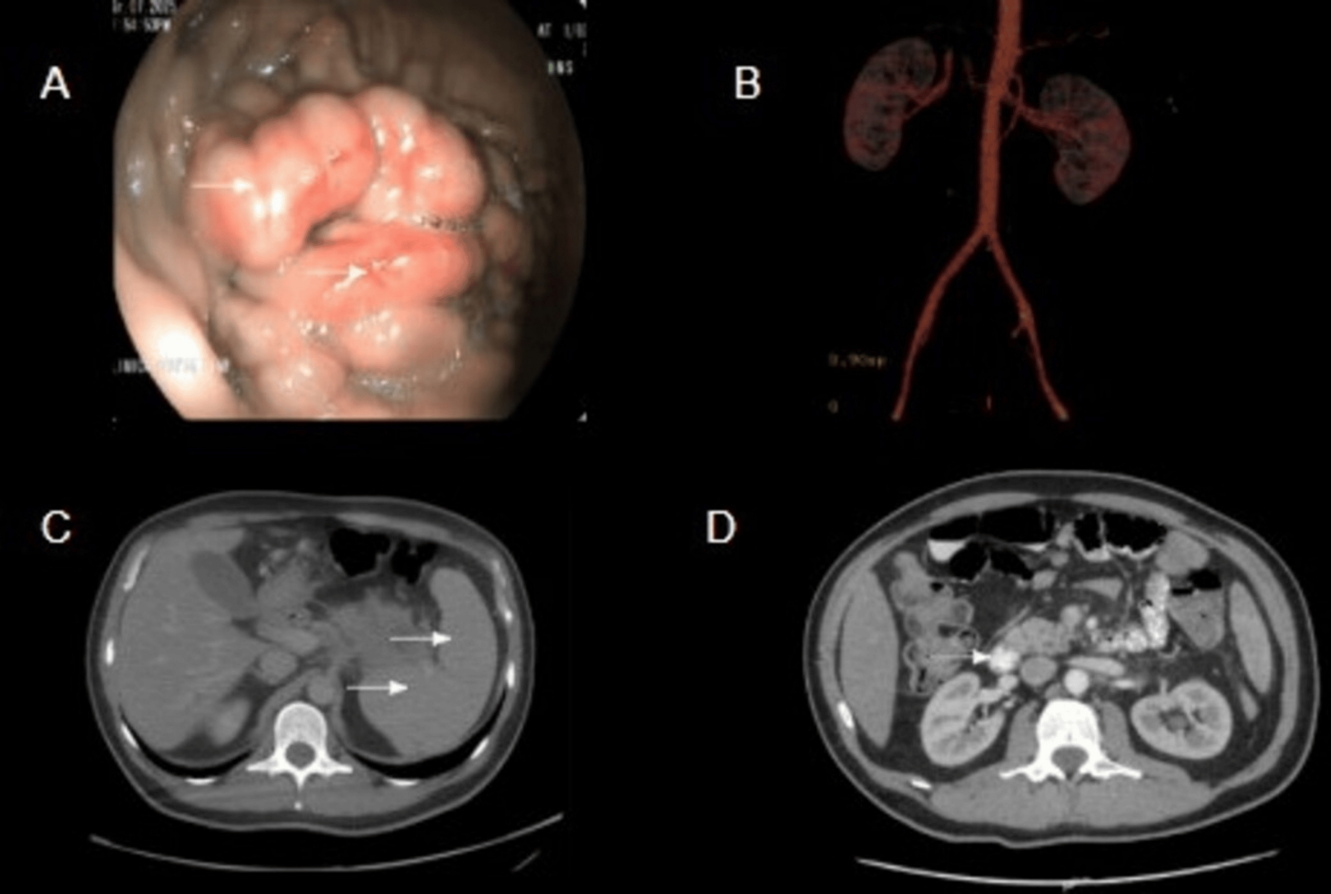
Multimodal imaging findings (A) EGD: arrows showing IGV1. (B) Angiography demonstrating a large gastrorenal shunt (outflow) directed toward the left renal vein. (C) Abdominal enhanced CT scan in portal venous phase: arrows showing dilated portal vein and splenomegaly. (D) Abdominal enhanced CT: arrow showing a hypodense pancreatic lesion. EGD: esophagogastroduodenoscopy, IGV1: isolated gastric varices type 1, CT: computed tomography
